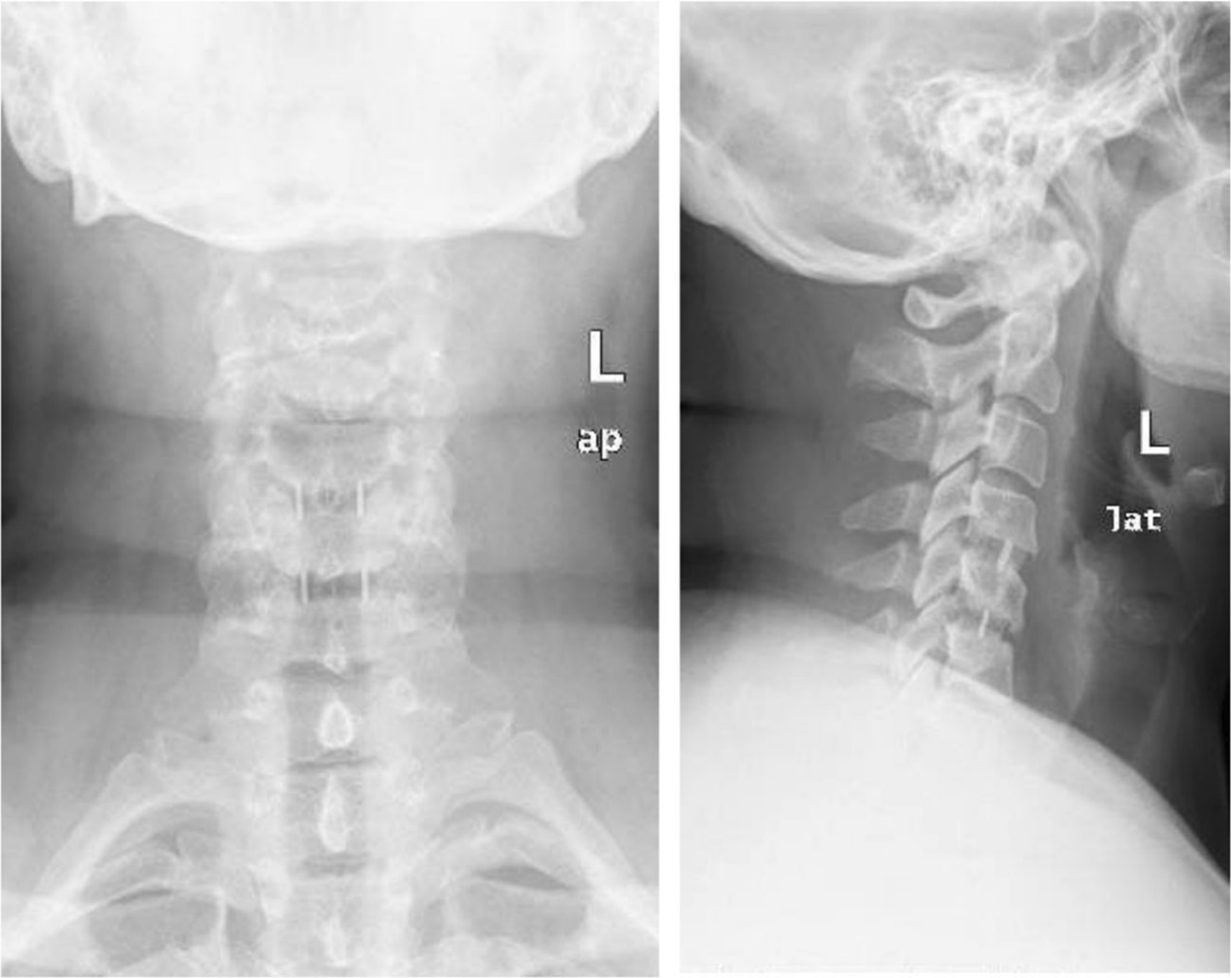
Post-operative radiographs of a 46-year-old female who underwent anterior cervical discectomy and fusion with PEEK cages filled with bone marrow aspirate. Surgical indication was right arm radiculopathy that failed non-surgical treatment
